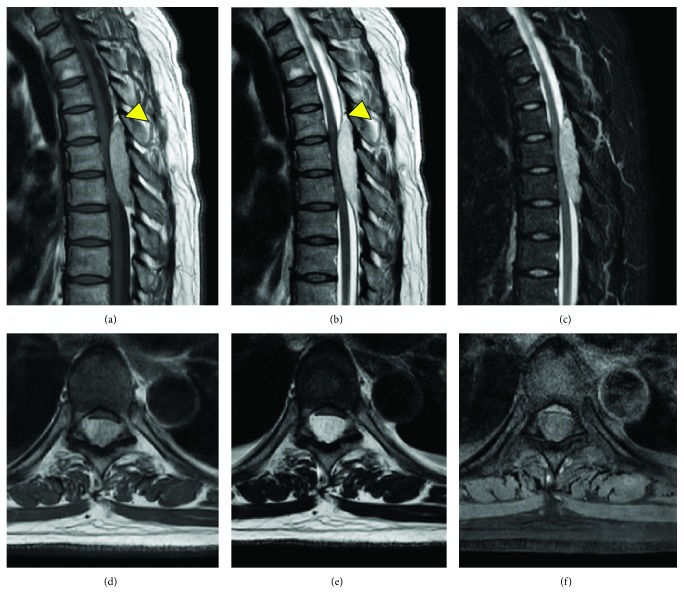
Preoperative thoracic MRI. A heterogeneous epidural posterior mass compressing the thoracic cord between Th5 and Th8 was revealed at admission. Sagittal view of the heterogeneous isointense mass of the T1-weighted image (a), heterogeneous hyperintense mass of the T2-weighted image (b), and enhanced mass of the fat-suppression image by short-T1 inversion recovery (STIR) (c). Axial view of the epidural mass of the T1-weighted image (d), T2-weighted image (e), and STIR image (f). This tumor had lipomatosis in its upper segment (arrowhead).
